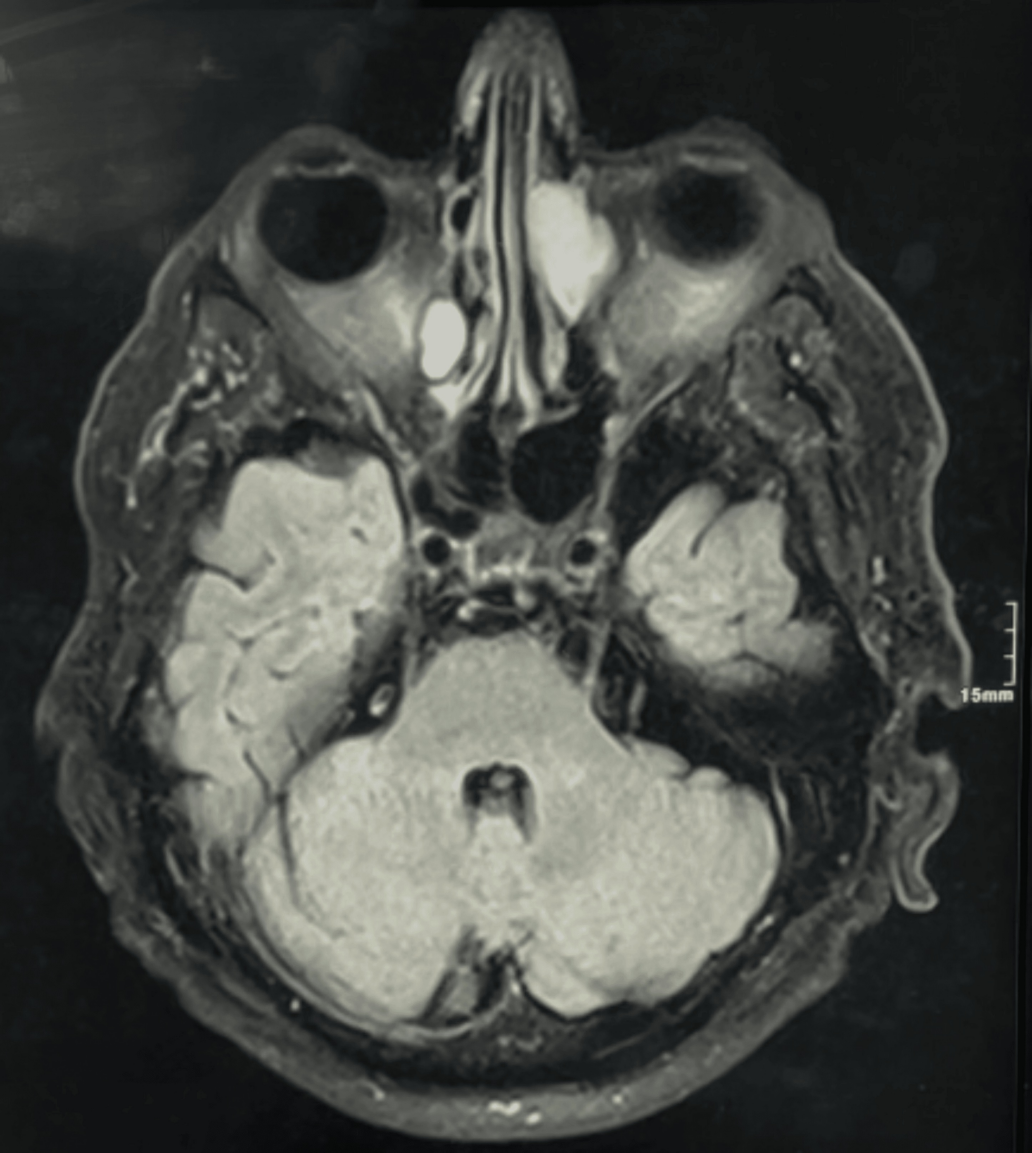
Preoperative cerebral MRI A 14 x 9 mm nodular filling of the adjacent right lamina papyracea. MRI: magnetic resonance imaging
